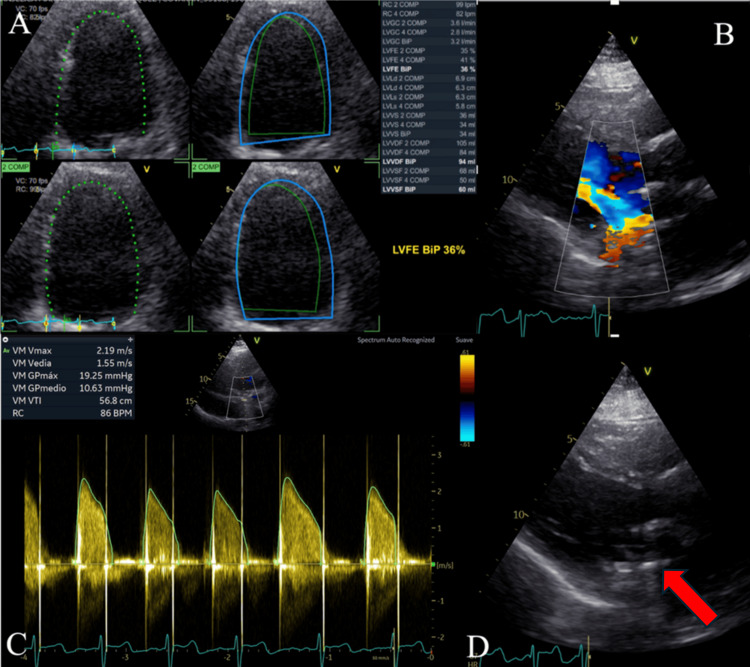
Transthoracic echocardiographic assessment of prosthetic mitral valve thrombosis. Panel A demonstrates quantitative left ventricular systolic function assessment by the Simpson biplane method, showing reduced left ventricular ejection fraction (LVEF, 36%). Panel B shows color Doppler interrogation across the mitral bioprosthesis, demonstrating turbulent transmitral flow. Panel C demonstrates continuous-wave Doppler across the prosthetic mitral valve, revealing elevated transprosthetic gradients (mean gradient, 10.63 mmHg; peak gradient, 19.25 mmHg) and increased transvalvular velocity (Vmax: 2.19 m/second), findings suggestive of prosthetic valve dysfunction. Panel D shows transthoracic echocardiography demonstrating restricted mobility of the anterior prosthetic hemi-disk (red arrow), consistent with prosthetic valve thrombosis.
